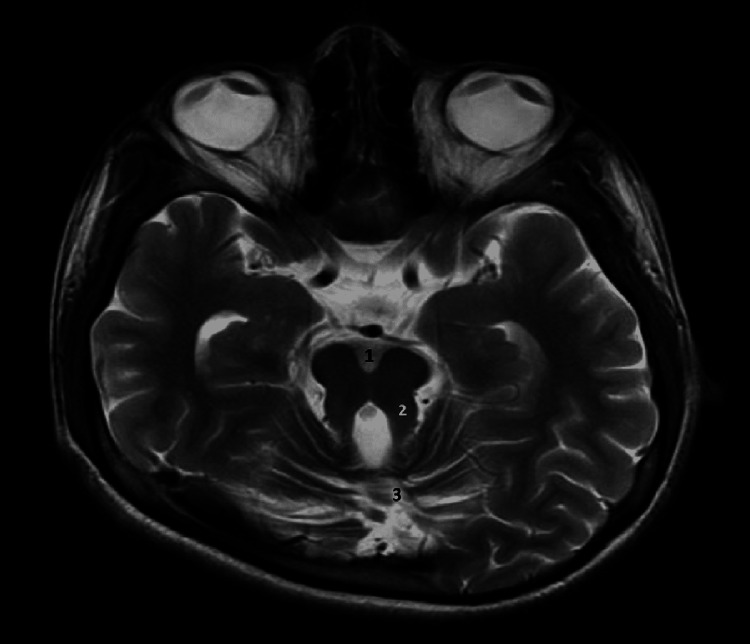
T2-weighted brain MRI in axial view showing deep interpeduncular fossa, elongated bilateral superior cerebellar peduncles, and hypoplasia of the cerebellar vermis, giving a molar tooth sign (MTS), consistent with Joubert syndrome
